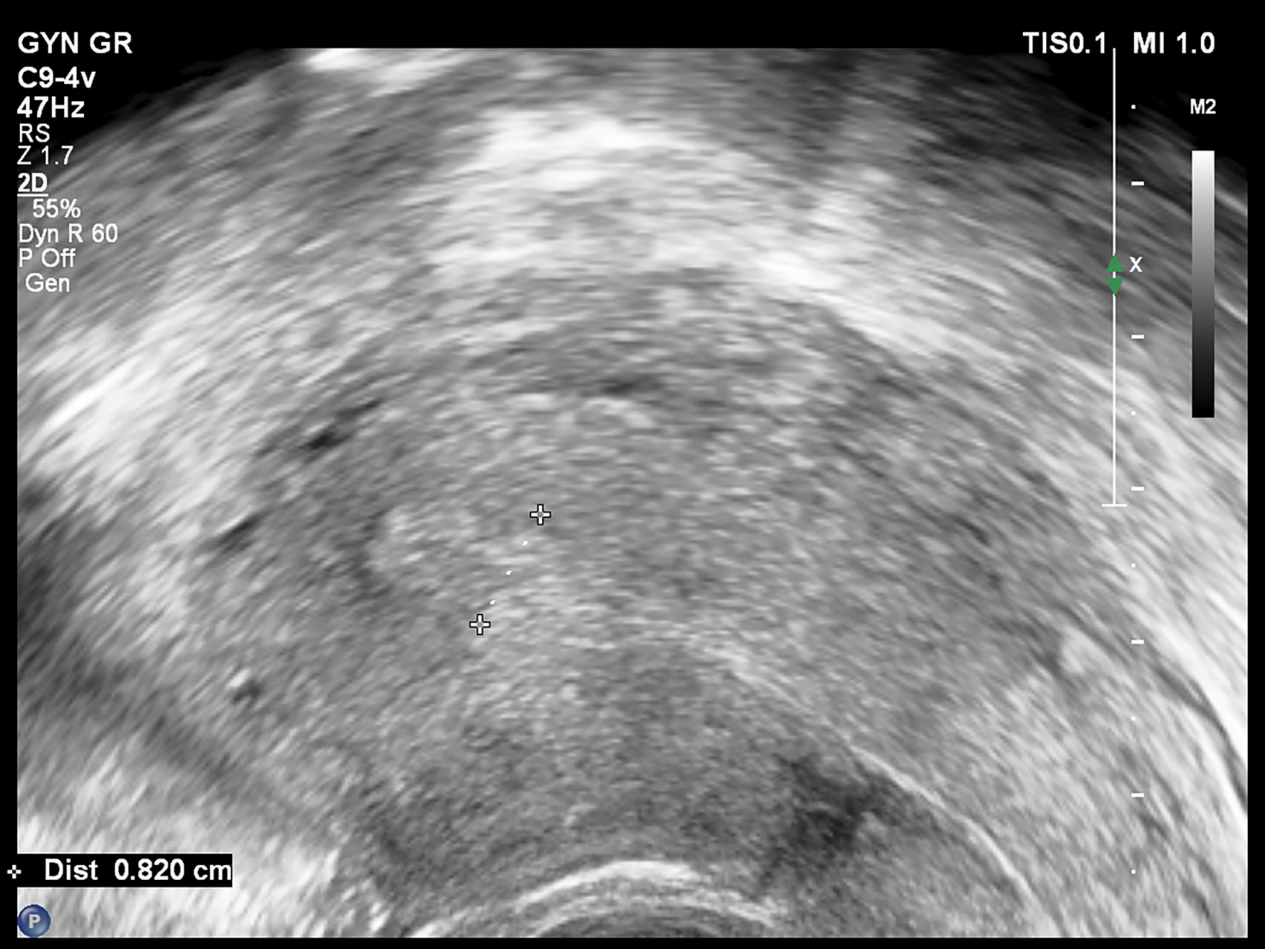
Follow‐up transvaginal ultrasound examination 17 days after hysteroscopic resection of the cornual pregnancy. The ultrasound confirms the absence of any retained products of conception and corroborates the findings from the sequential, diminishing β‐hCG levels.
